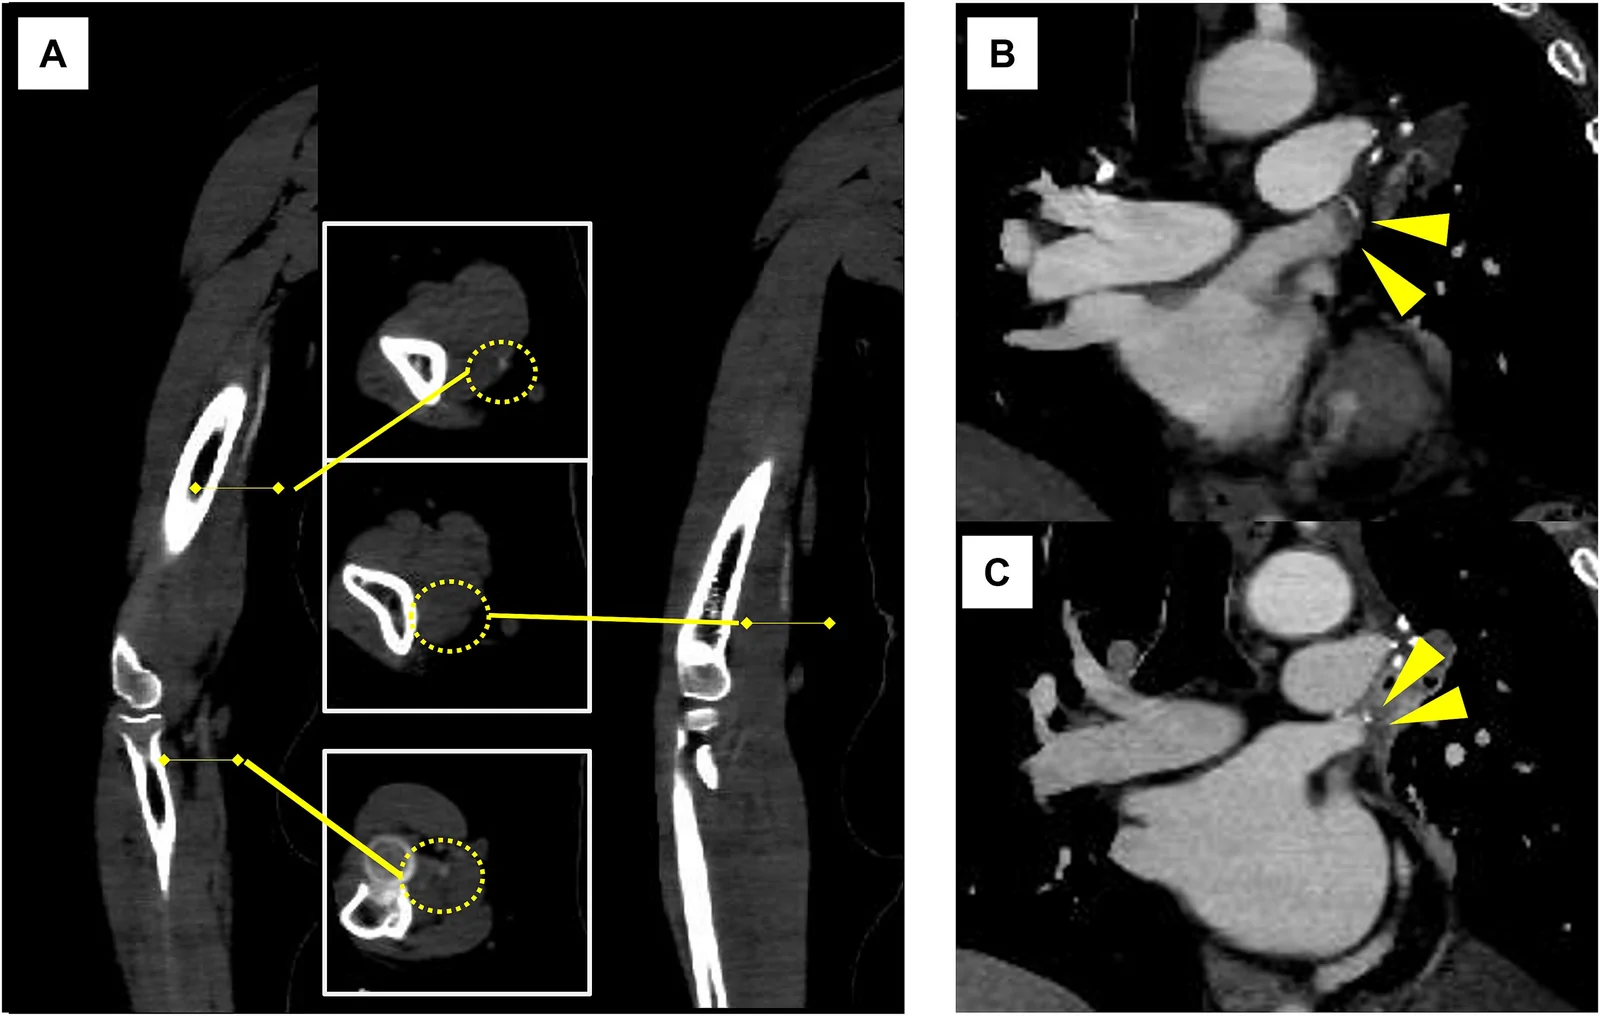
Contrast-enhanced CT findings at the onset of right brachial artery embolism and at follow-up. (A) Contrast-enhanced CT shows occlusion of the right brachial artery near the distal humerus, with collateral opacification of the radial and ulnar arteries distal to the occlusion. (B) Contrast-enhanced CT performed immediately after the onset of right brachial artery embolism on postoperative day 2 shows a small filling defect near the left upper-division pulmonary vein stump, suggestive of pulmonary vein stump thrombosis after left upper division segmentectomy. (C) Contrast-enhanced CT on postoperative day 28 shows resolution of the filling defect, with no residual thrombus in the left upper-division pulmonary vein stump.
